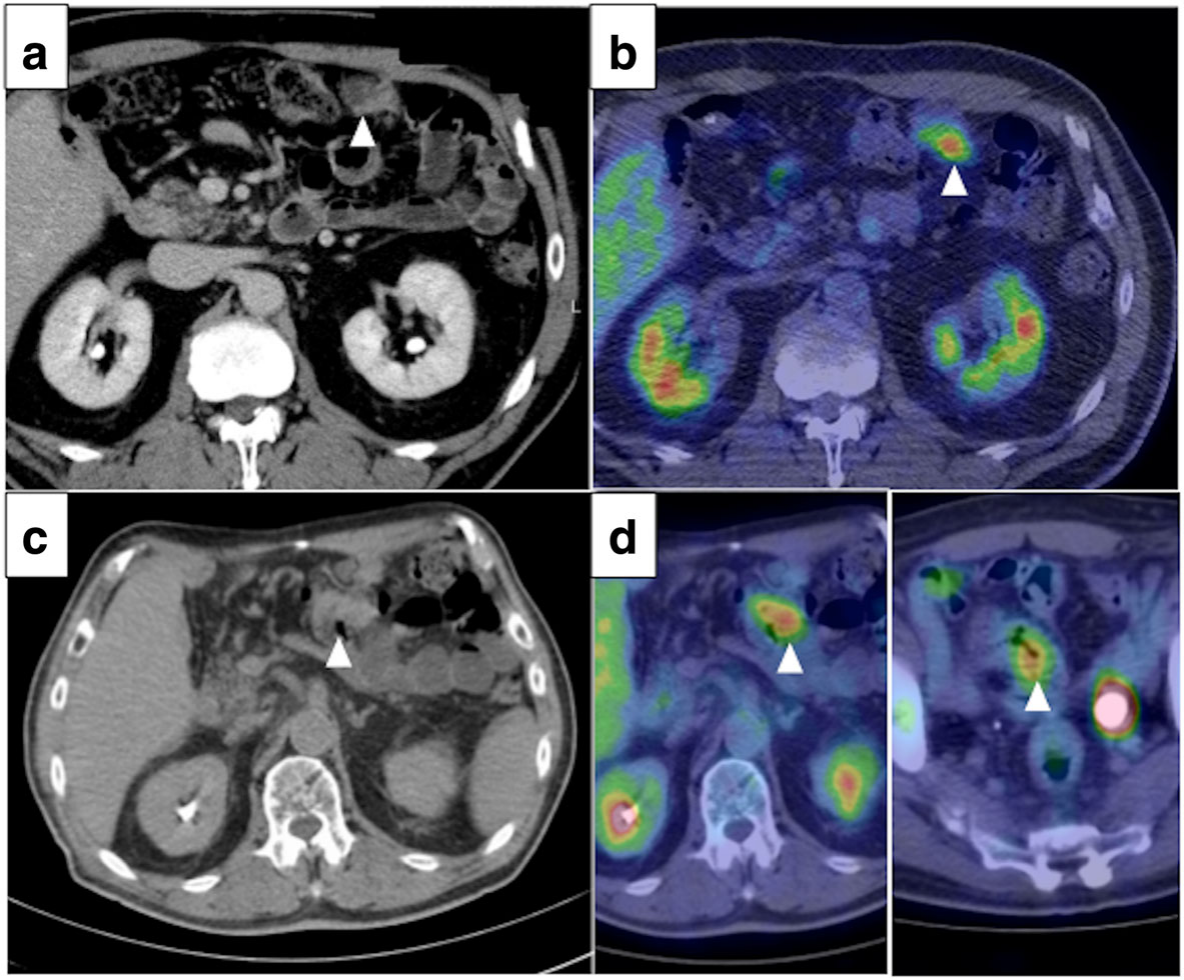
Recurrence of peritoneal dissemination. Peritoneal dissemination was detected by CT (a) and positron emission tomography-CT (PET-CT) (b). Bowel obstruction by the tumor was detected by CT (c) and PET-CT (d). Arrowheads show the peritoneal disseminations
